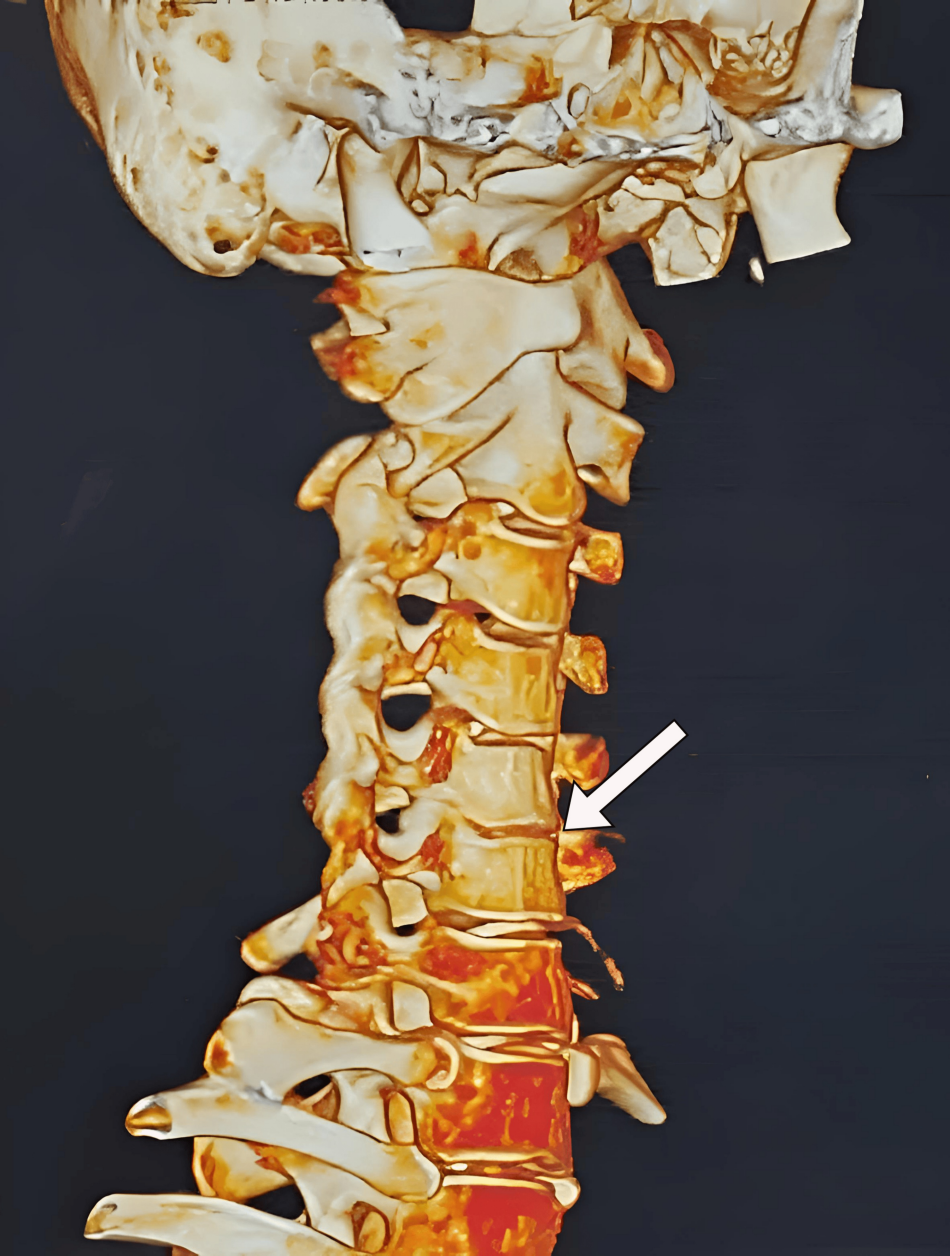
3D CT of the cervical spine. The three-dimensional computed tomography (CT) of the cervical spine showing loss of cervical lordosis and decreased intervertebral space (white arrow) between the C5-C6 level.
